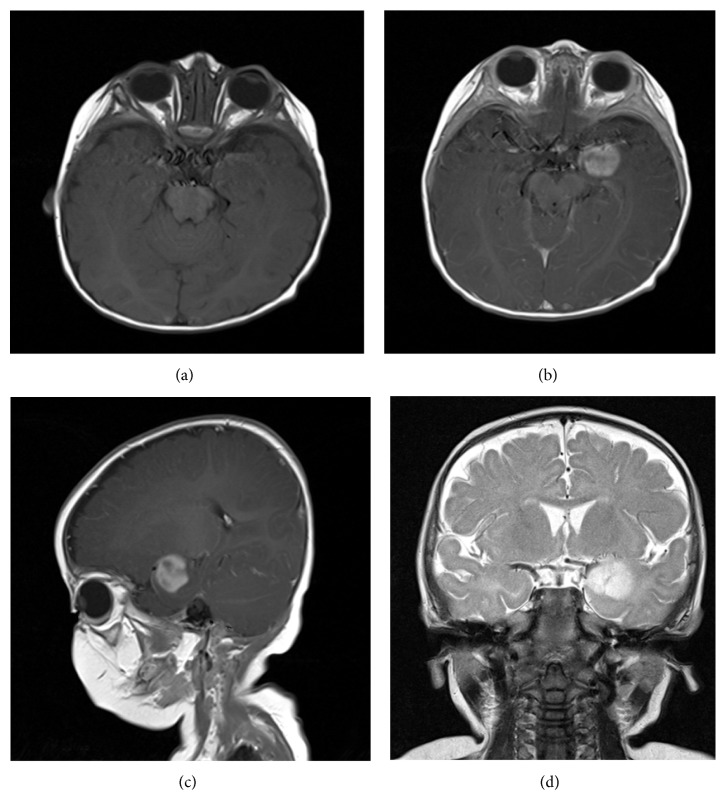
(a) Axial T1 precontrast, (b) axial T1 postcontrast, (c) sagittal T1 postcontrast, and (d) coronal T2 magnetic resonance imaging show a heterogeneously enhancing mass in the left temporal lobe.
